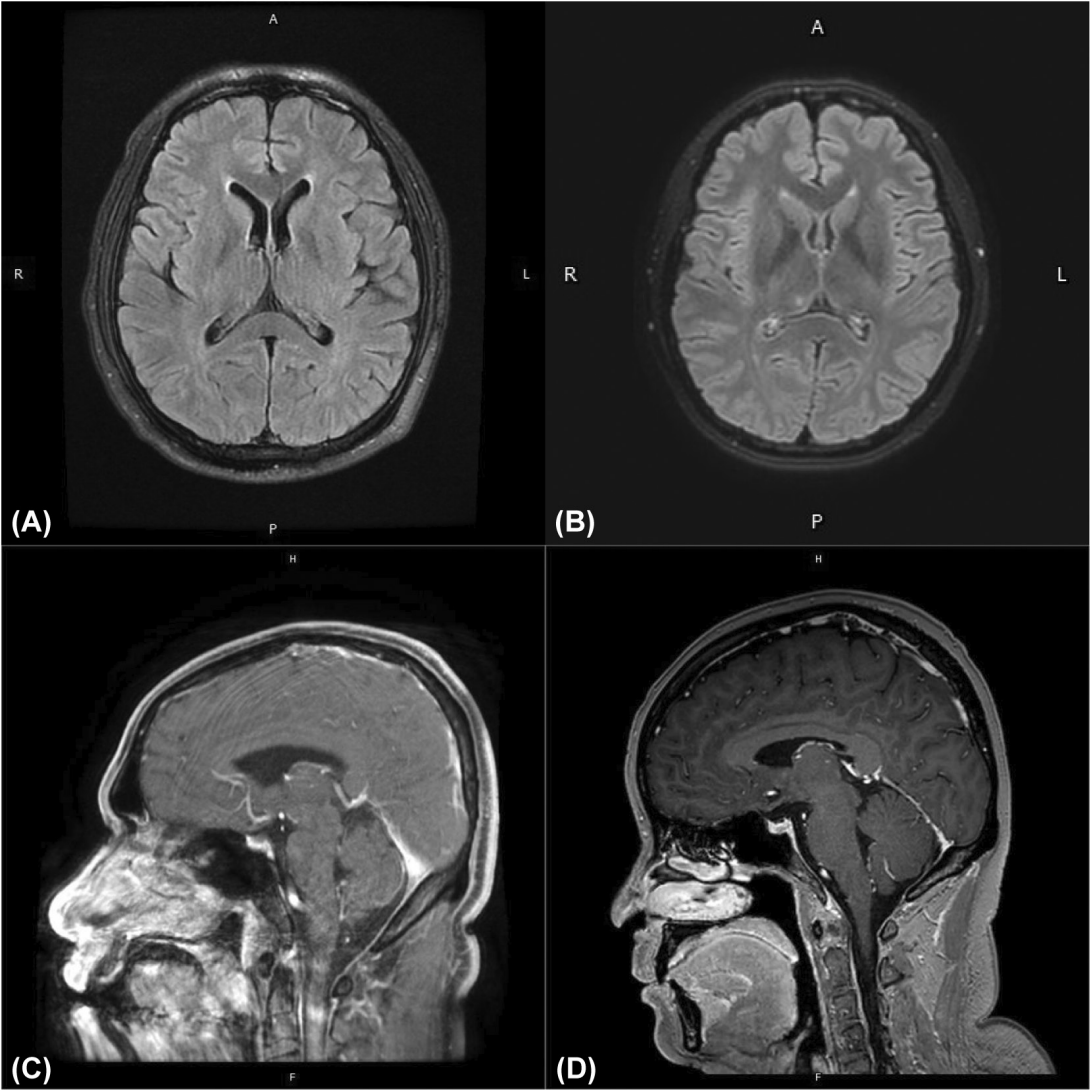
(A) T2‐FLAIR images of the brain MRI of patient 1 after AE onset showed no abnormal hyperintensity. (B) T2‐FLAIR images of the brain MRI of patient 2 after AE onset showed no abnormal hyperintensity. (C) Lack of enhancement of the brain MRI of patient 1 after AE onset. (D) Lack of enhancement of the brain MRI of patient 2 after AE onset. (Brain MRI images of patient 3 after AE onset cannot be accessed but its word report showed no abnormal hyperintense.) More images can be found in Figure S2.
